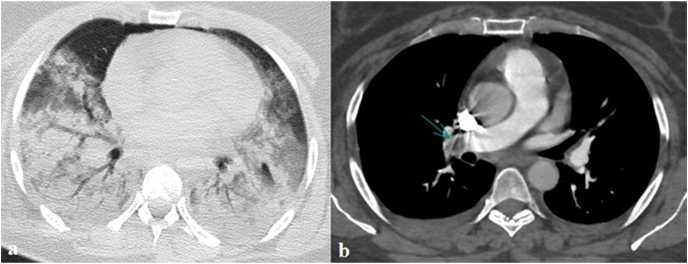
a: Axial lung window showing multiple confluent ground-glass opacities and consolidative areas predominantly in the lower lobes. SARS-Cov-2 RT-PCR positive, CT findings consistent with COVID-19 pneumonia CO-RADS 6. b: Axial C + CTPA showing a filling defect seen within the distal right main pulmonary artery (right proximal pulmonary embolism).
